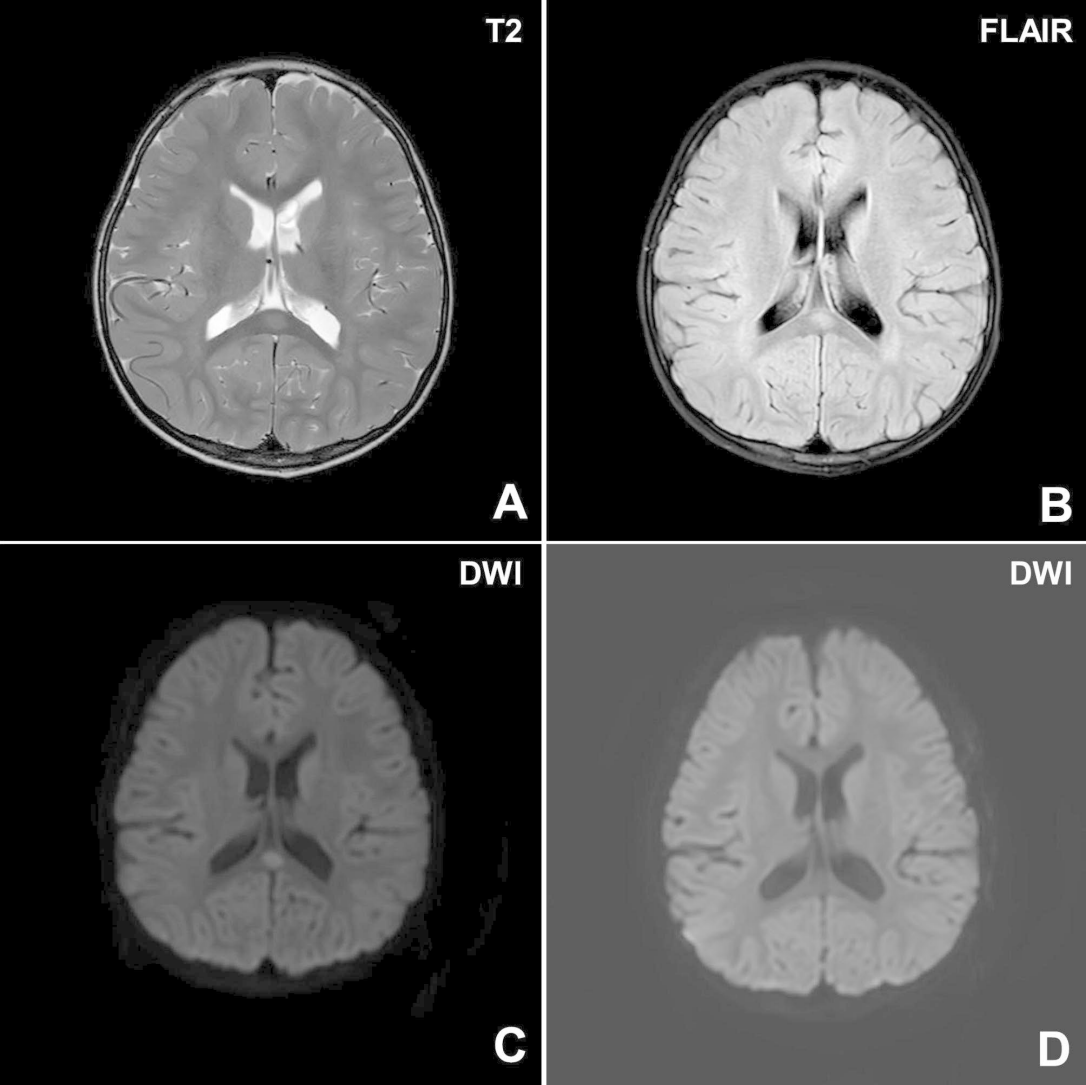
Brain magnetic resonance imaging (MRI) at the time of diagnosis (A–C) and 47 days after the first visit (D) in patient 2. Axial T2-weighted (A) and fluid-attenuated inversion recovery (FLAIR, B) images showed a 1-cm hyperintense lesion in the splenium of the corpus callosum. This lesion with diffusion restriction (C) on diffusion imaging at the time of diagnosis was not detected in the corpus callosal splenium 47 days after the first visit (D)
